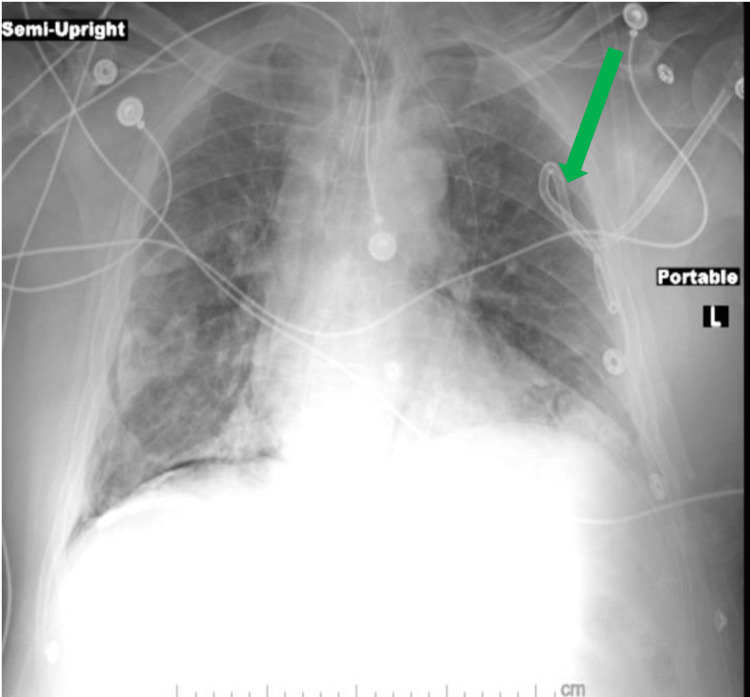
Chest radiograph, with left-sided chest tube in place and improvement of pneumothorax. Green arrow chest tube. A persistent right pneumothorax that did not require decompression.
